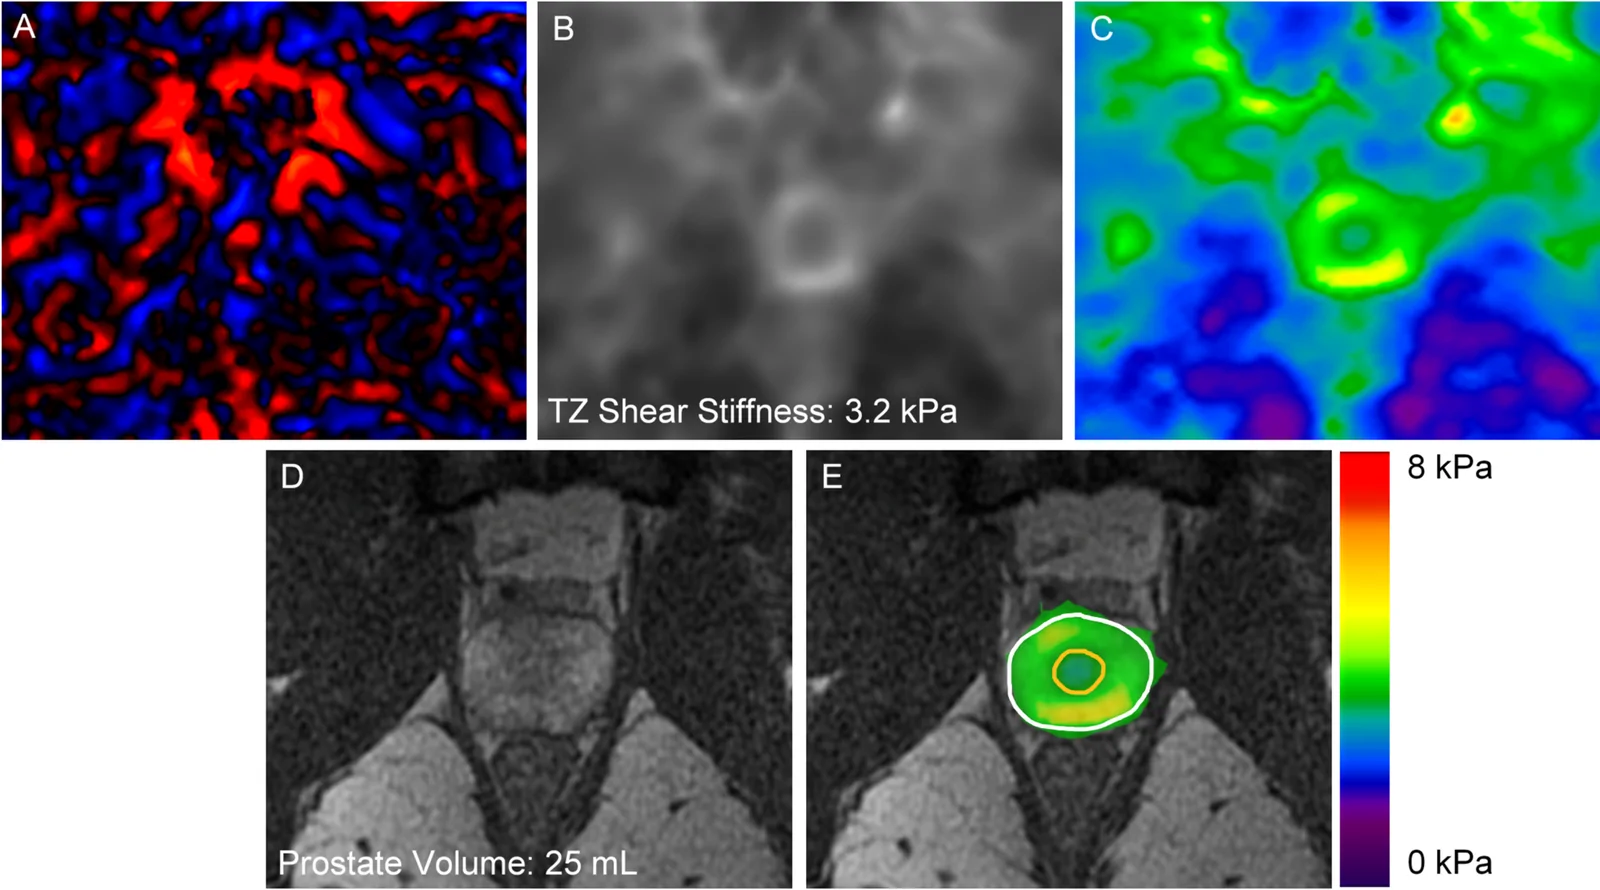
Magnetic resonance imaging (MRI) and 3D vector prostate magnetic resonance elastography (pMRE) of a 40-year-old man without benign prostate hyperplasia/lower urinary tract symptoms (BPH/LUTS). Axial wave image (A), shear stiffness (B) and color maps (C), T2-weighted (T2w) image (D) and fusion of shear stiffness color map on T2w image (E) of the prostate, at the midgland. On the fusion image, the prostate is outlined in white and the transition zone (TZ) in yellow. The mean prostate TZ shear stiffness was 3.2 kPa. Notice the stiffness of the TZ is lower than the peripheral zone in the asymptomatic man
